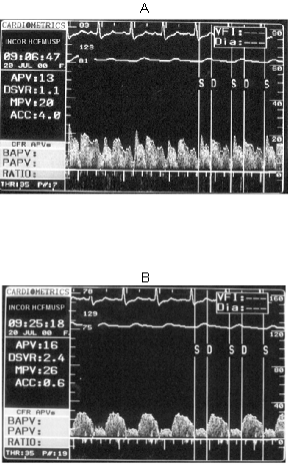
Baseline spectral Doppler coronary flow velocity signal in right coronary artery (A) and left anterior descending coronary artery (B). S = systolic, D = diastolic, portions of phasic coronary flow. APV = time-averaged peak coronary flow velocity. DSVR = diastolic/systolic flow velocity ratio.
